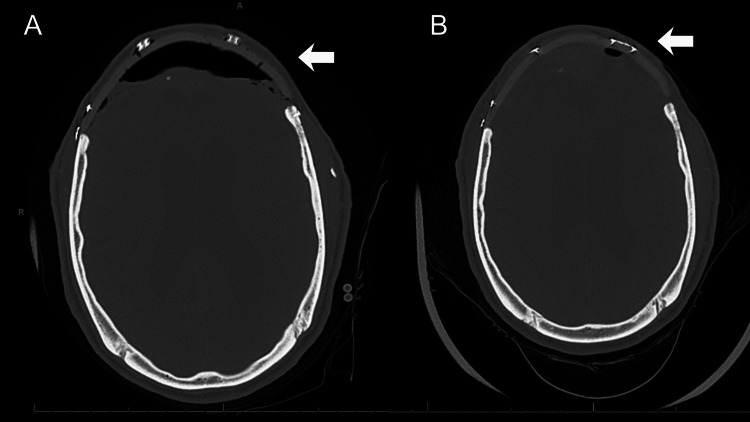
Cranial CT scan (A) Pneumocephalus identified after a postoperative decrease in the level of consciousness. (B) Follow-up imaging showing reduction of pneumocephalus 12 days post surgery.
